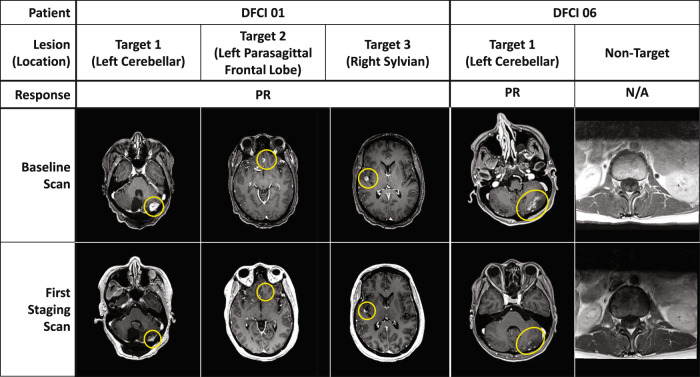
Radiographic responses to progressive HER2 + LM treated with T-DXd. Baseline and LM response assessment by MRI Brain imaging of two patients with HER2+ LM treated with T-DXd. Encircled are unirradiated CNS lesions highlighting response.
